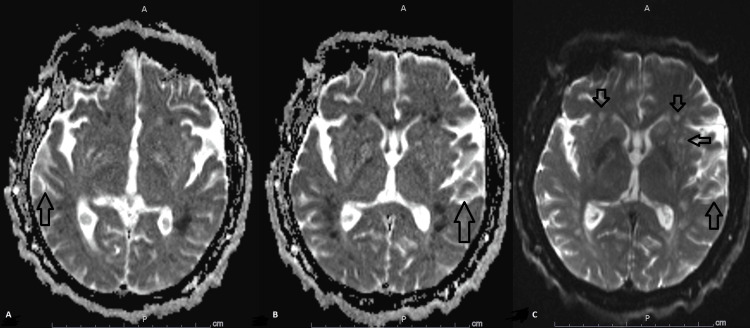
Diffusion trace MRI brain Diffusion trace MRI brain sequence showing the progression of cerebral infarctions (arrows in A, B, and C) and bilateral centrum semiovale, corona radiata, periventricular and gangliocapsular areas as well as corpus callosum (arrows in C)
